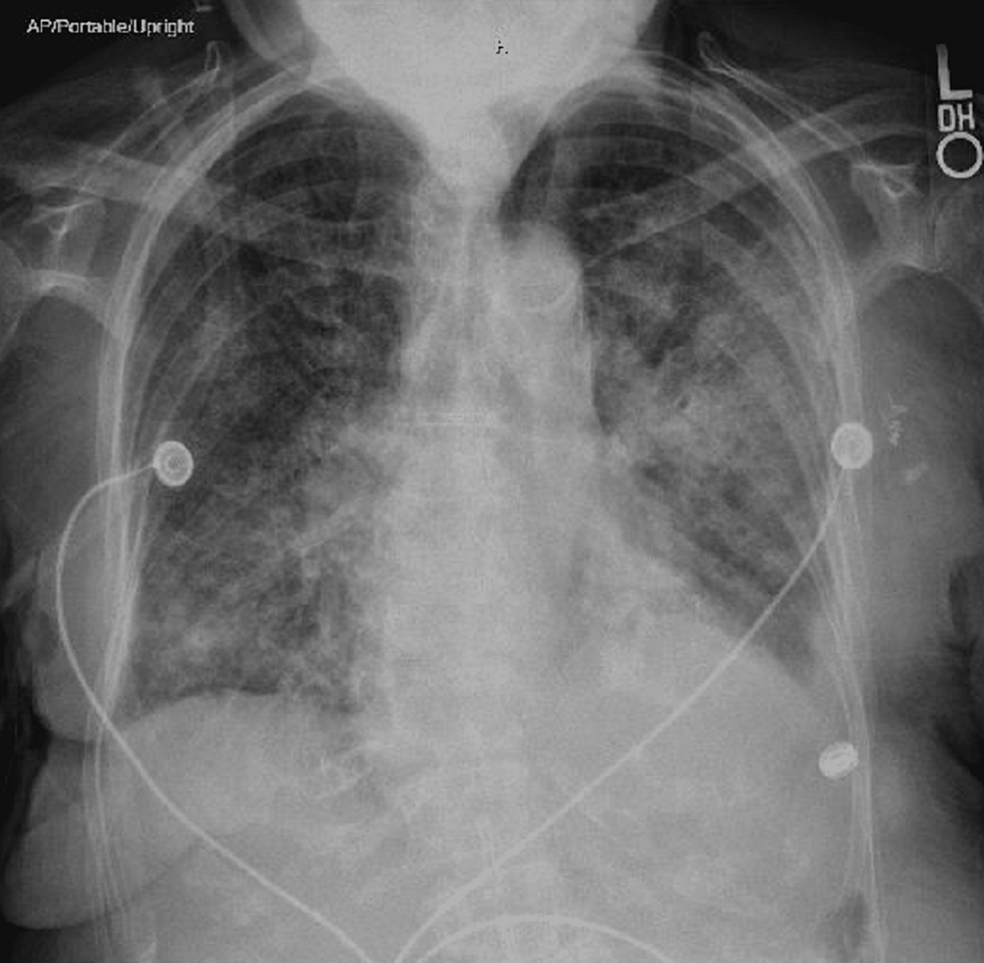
Chest X-ray showing diffuse multifocal interstitial opacification with cardiomegaly and pulmonary edema
